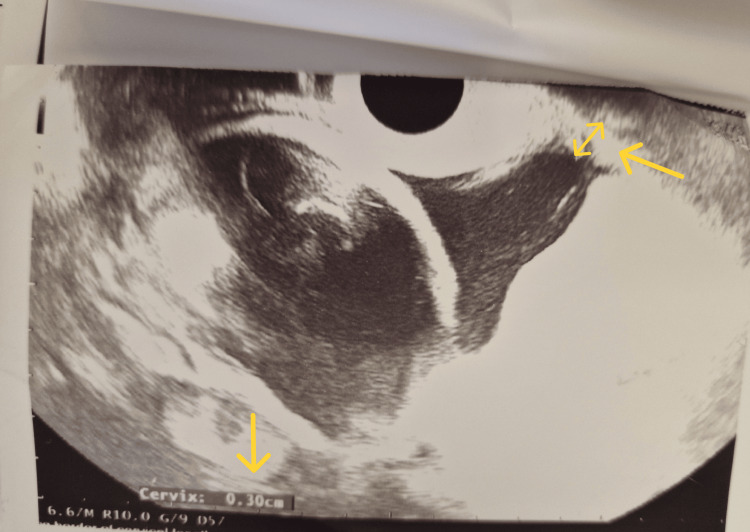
Transvaginal ultrasound at 23 weeks revealing funneling and shortening of cervical length (arrows)
